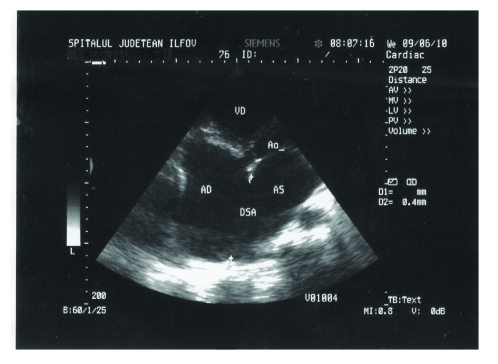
2D Transthoracic echocardiography. Atrial septal defect in apical 4 chamber–view. AD=right atrium, AS=left atrium, VD=right ventricle, A0=aorta, DSA=atrial septal defect.
